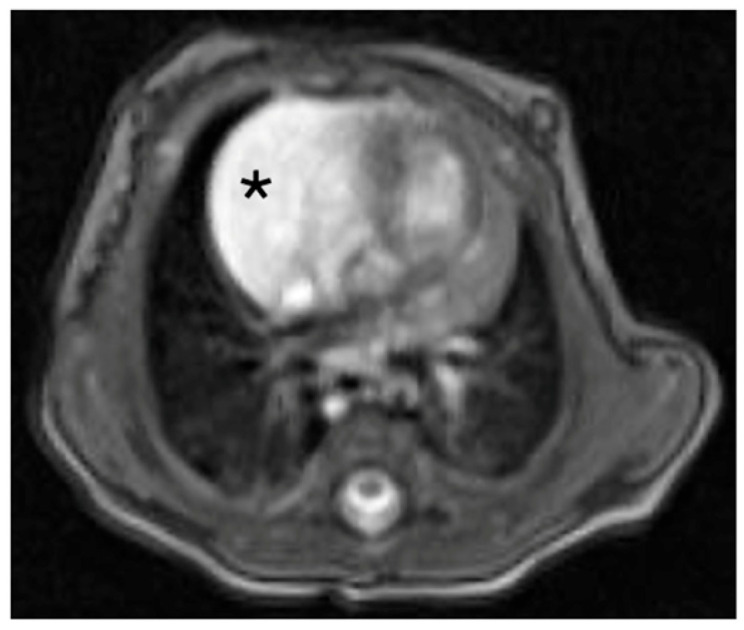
MRI T2-weighted. Right paracardiac mass with well-defined margins, showing hyperintense signal on T2-weighted images and containing some branching inhomogeneities suggestive of a possible vascular nature. Asterisk: lesion.
